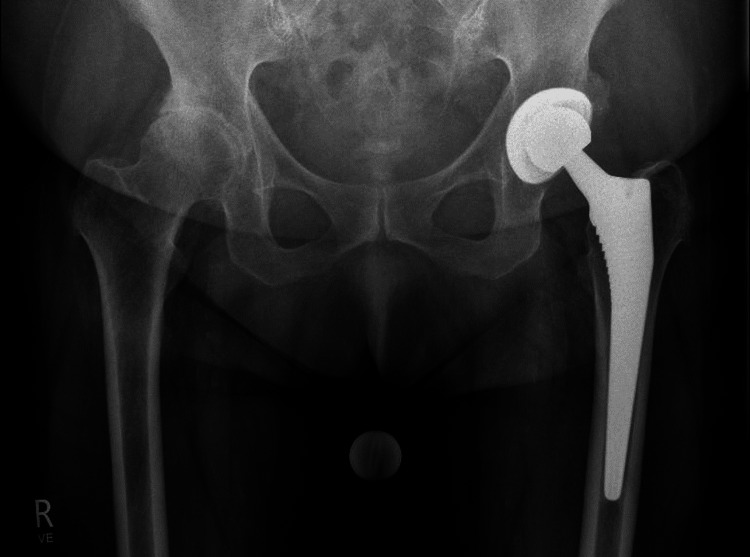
Preoperative anteroposterior radiograph of the pelvis showing advanced osteoarthritis of the right hip with joint space narrowing, subchondral sclerosis, and osteophyte formation. The left total hip arthroplasty, performed 10 years earlier, appears well-fixed with no signs of loosening.
